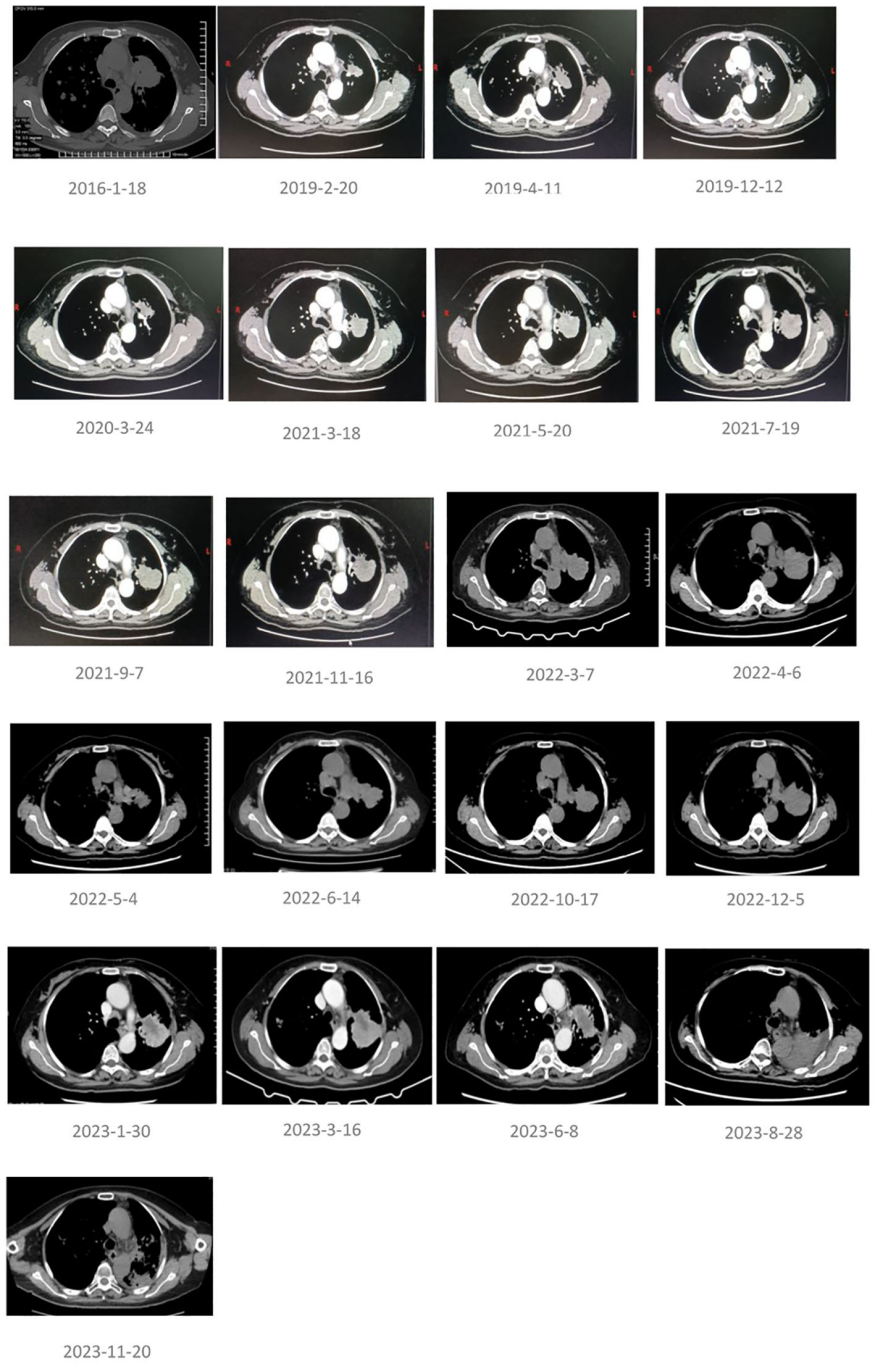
Chest CT images at key points during the patient’s treatment process.
